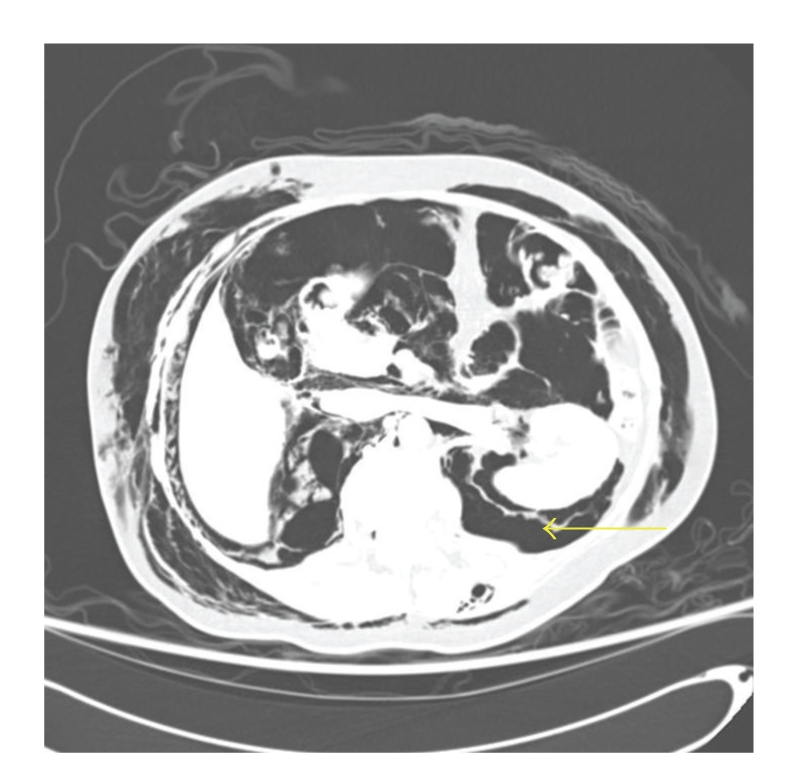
Transverse section of CT abdomen showing subcutaneous emphysema, pneumoperitoneum, and pneumoretroperitoneum (arrow).
